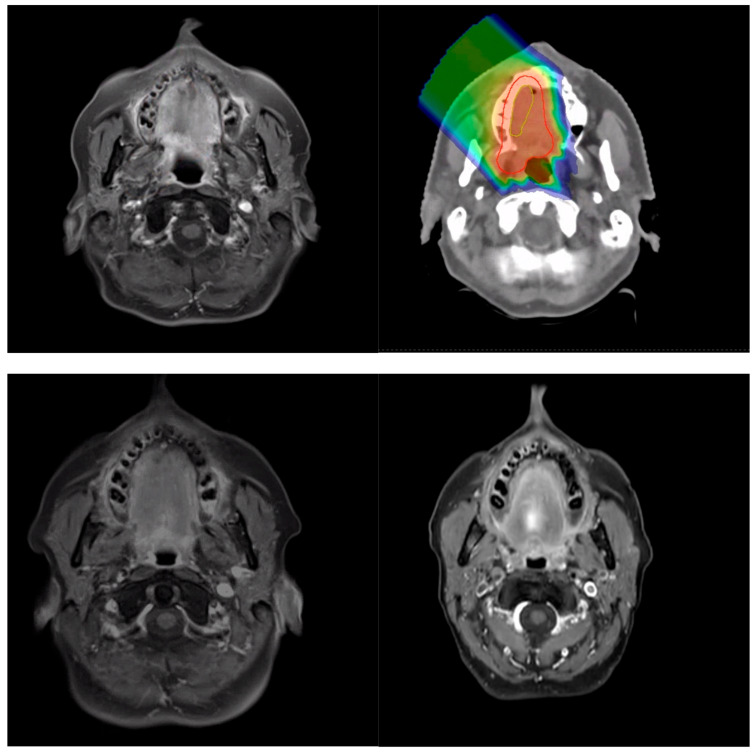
Radiologic tumor response following treatment. Tumor size changes at 12, and 24 months are shown relative to baseline imaging. Representative images from a single patient include the pre-treatment MRI and the corresponding radiotherapy plan, demonstrating target volume delineation and dose distribution.
